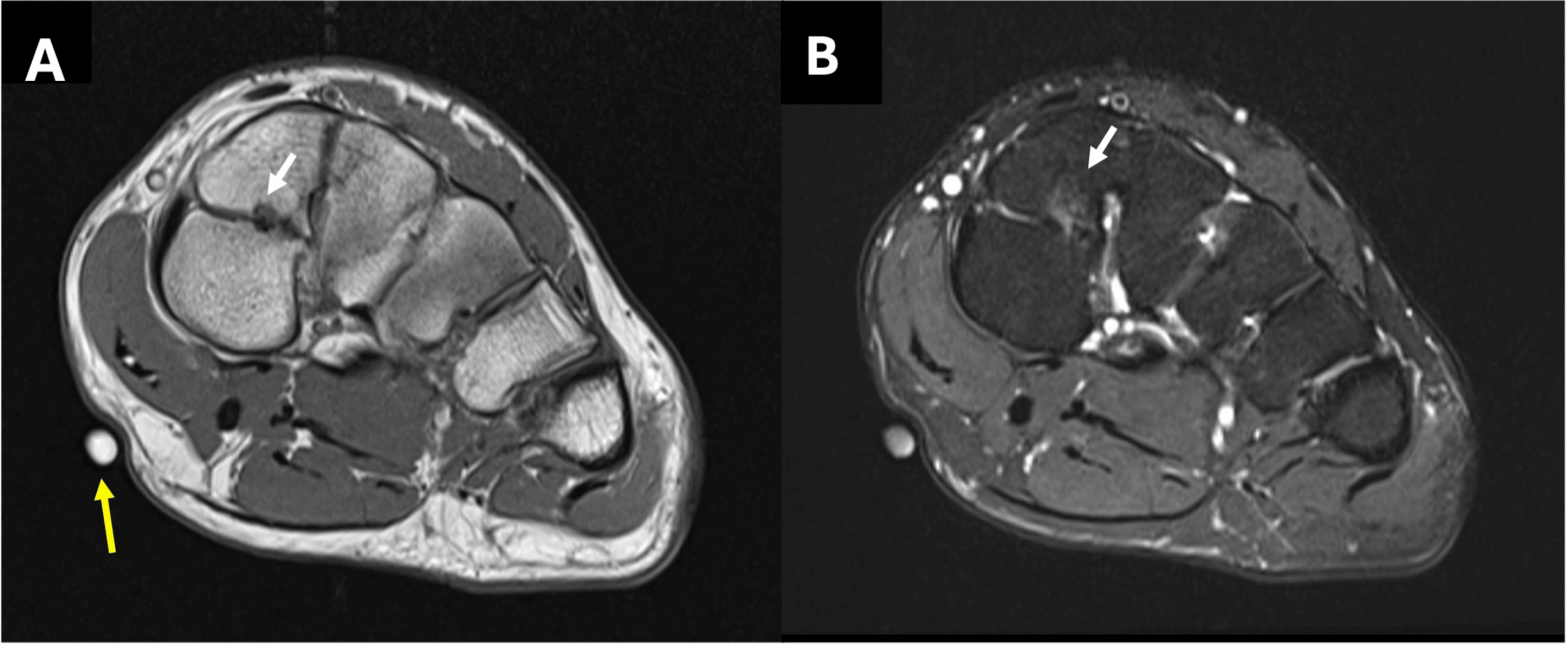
Short-axis T1 MRI (A) and PD FS MRI (B) showing dorsal and plantar components of bipartite medial cuneiform with associated pseudoarthrosis and degenerative changes (white arrows). The marker (yellow arrow) is noted on the medial plantar aspect of the foot directly under the medial cuneiform. PD FS MRI: proton density fat saturation MRI
